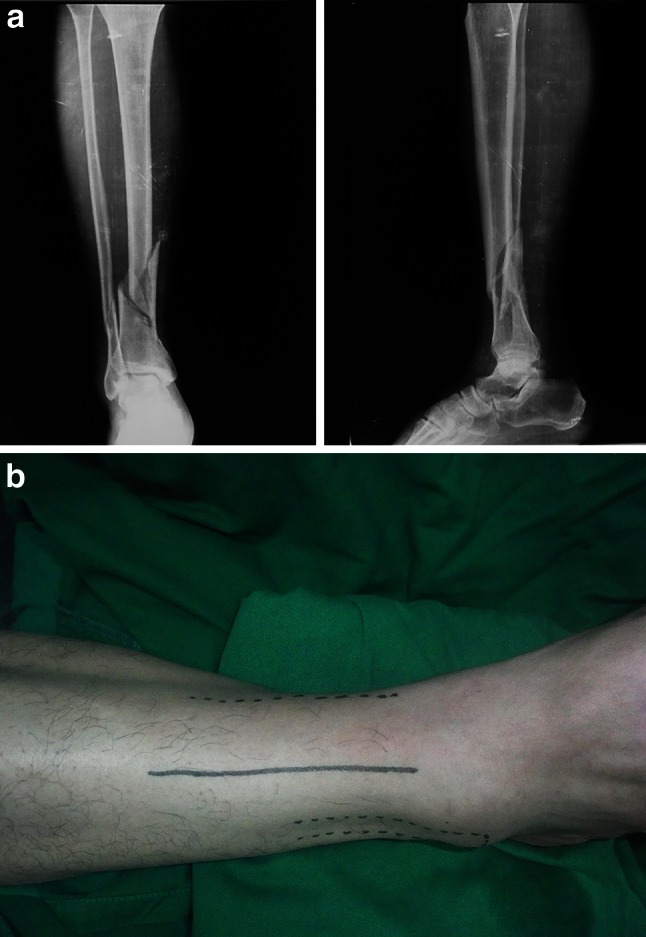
a X-ray of a 42-C1 fracture of the right leg. b Superficial markings of prominent anatomic features for the longitudinal incision made along the line of projection of the EDL muscle
